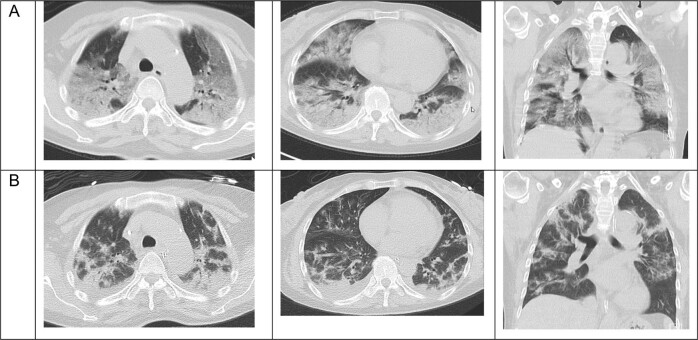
Ruxolitinib clears lung infiltration in SARS-CoV-2 induced ARDS. a Chest CT scans of the patient before transfer to the ICU reveals diffuse bilateral ground glass opacities, consolidations, and motion artifacts due to tachypnea. b After 11 days of treatment with ruxolitinib, markedly decreased ground glass opacities and motion artifacts, less pronounced regression of consolidations, and atelectasis.
